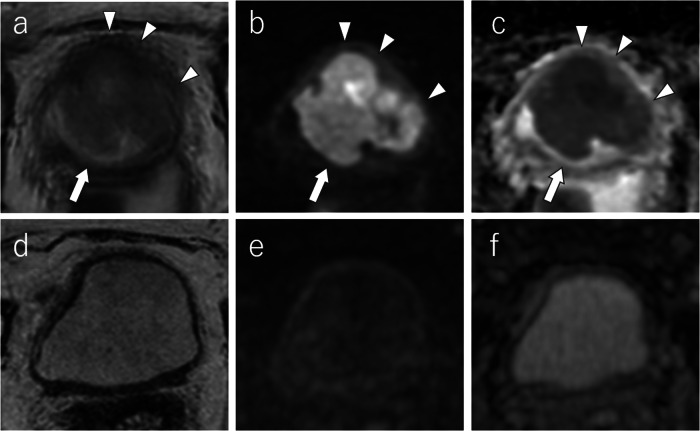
Trimodality therapy responder: a 74-year-old woman with a 53-mm urothelial carcinoma in the anterior bladder wall Pre-therapy MRI shows a lobulated tumor with partial disruption of the muscle layer on T2WI (a) [T2WI VI-RADS score: 4], high signal intensity on DW-MRI suggesting muscle infiltration (b) [DW VI-RADS score: 4], and homogeneous low signal intensity on the ADC map with a mean ADC value of 1.160 × 10^−3^ mm^2^/s (c). Post-therapy MRI, after trimodality therapy consisting of transurethral resection of bladder tumor (TURB), two courses of cisplatin treatment, and intensity-modulated radiation therapy (IMRT 60 Gy/30 fr), demonstrates significant tumor reduction with no apparent lesions on T2WI (d), DW-MRI (e), or the ADC map (f). The tumor is indicated by a white arrow, and disruptions or infiltrations by white arrowhead. ADC, apparent diffusion coefficient; DW-MRI, diffusion-weighted; IMRT, intensity-modulated radiation therapy T2-weighted imaging; TURB, transurethral resection of the bladder; VI-RADS, vesical imaging-reporting and data system
